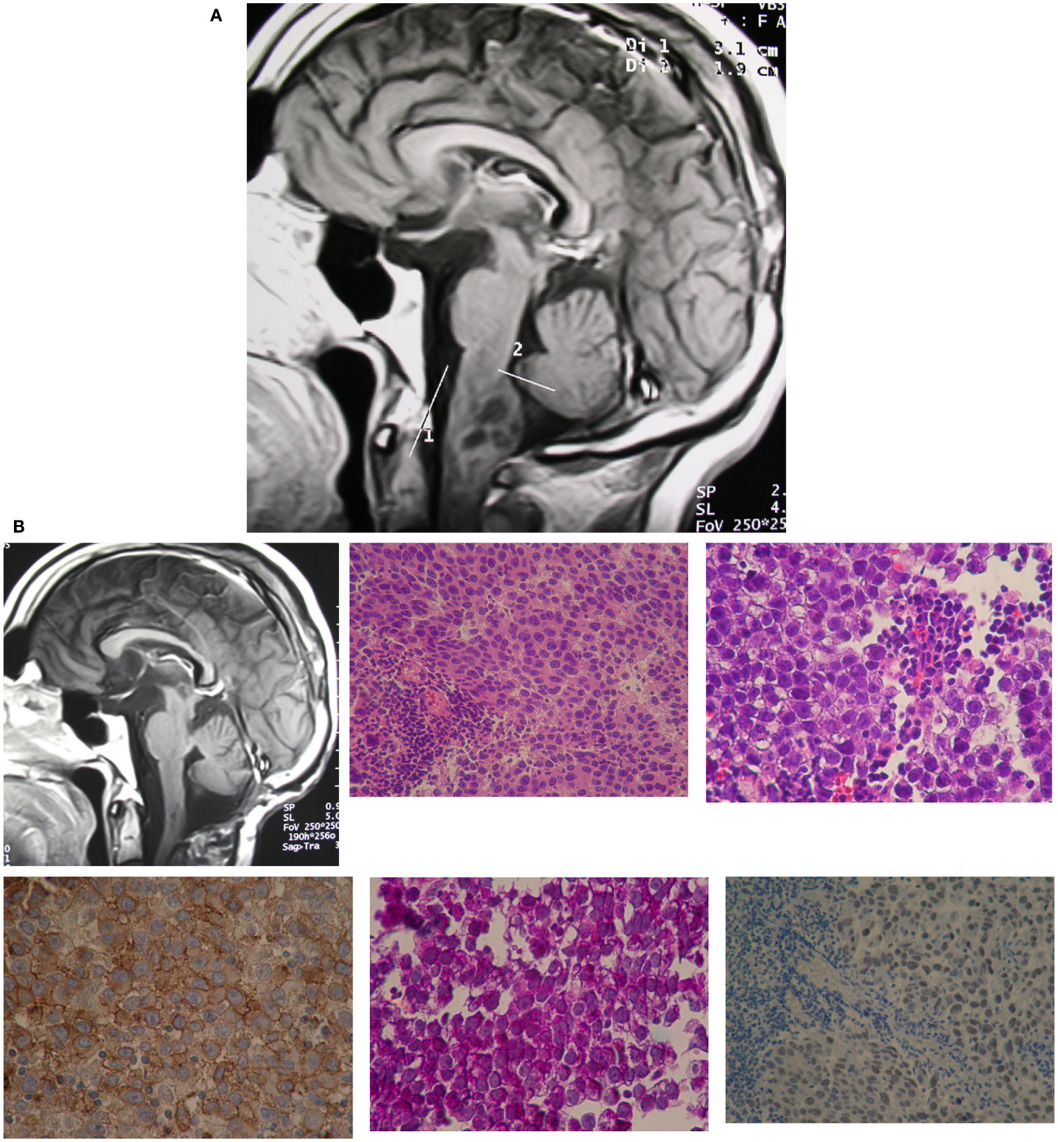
(A) Case 2. Preoperative T1-weighted MRI scans showed a wider MO due to a hypointense heterogeneous mass with necrosis or polimicrocystic component. Gadolinium-enhanced T1-weighted MRI scans demonstrated a heterogeneous enhancing mass. (B) T1-weighted brain MRI scans showed postoperative result.
